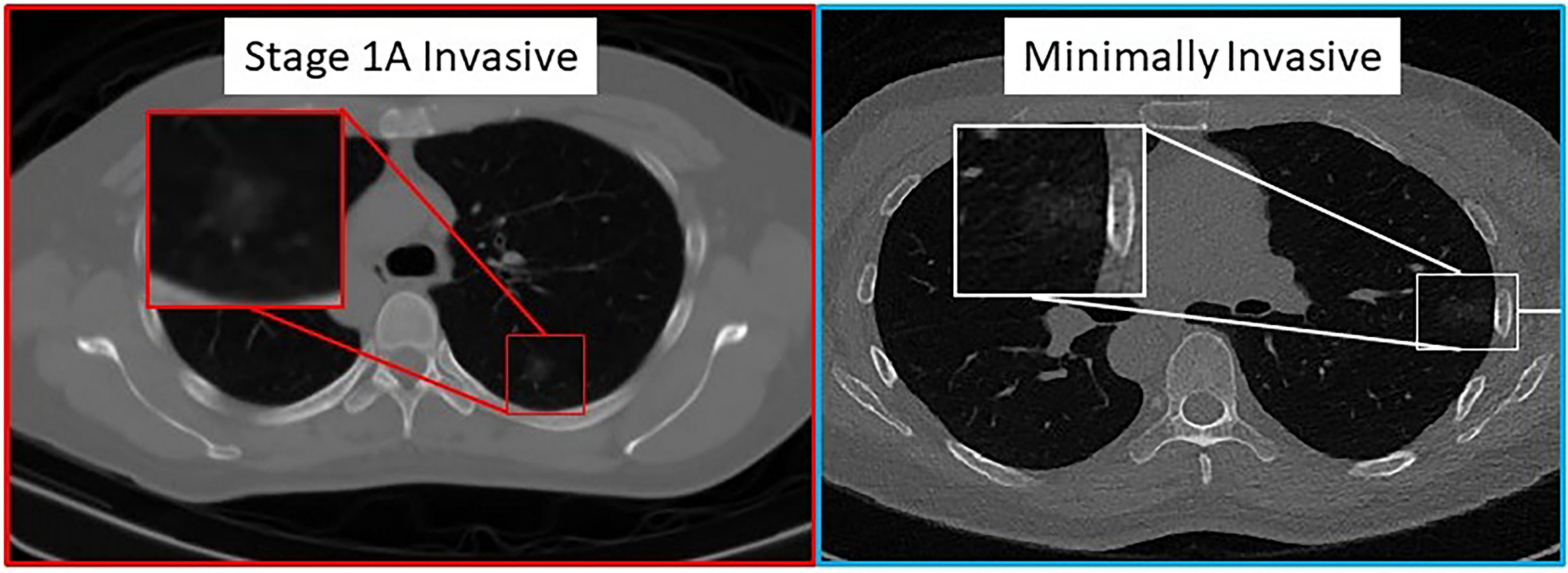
Pathologically proven INV (left image) and MIA (right image) cases presenting as predominantly ground-glass nodular densities which are indistinguishable on CT imaging.
